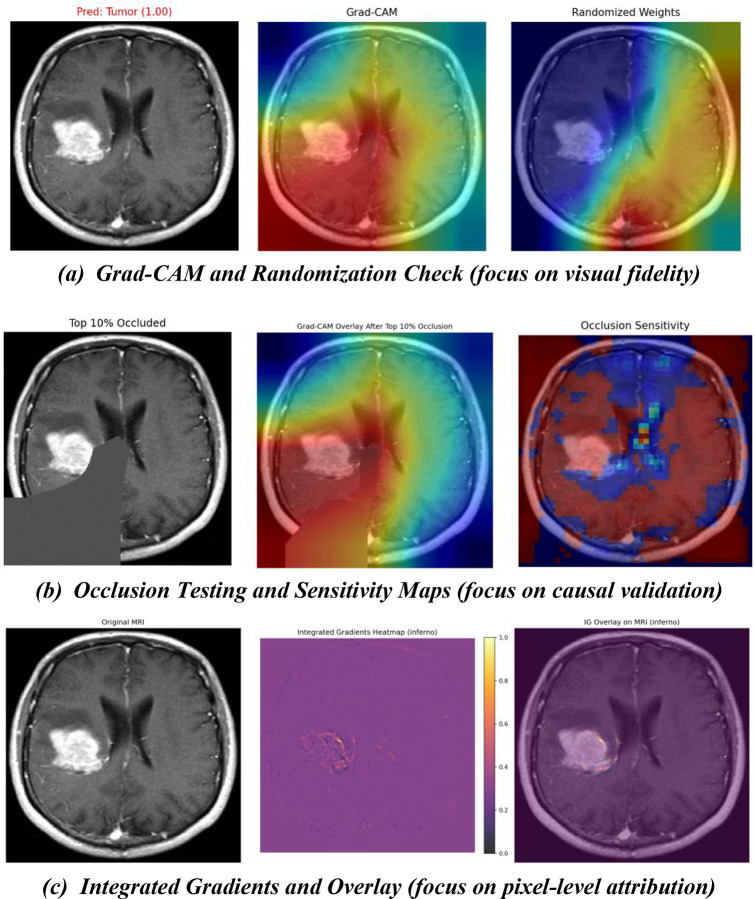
Representative Example of XAI Methods on Brain MRI Tumor Case from Internal Test Dataset. (a) Grad-CAM highlights tumor regions, while randomization disrupts activations. (b) Occlusion testing and sensitivity maps confirm causal relevance of highlighted areas. (c) Integrated Gradients overlays, visualized with a perceptually uniform colormap (Inferno), provide pixel-level attribution consistent with Grad-CAM, reinforcing interpretability reliability.
